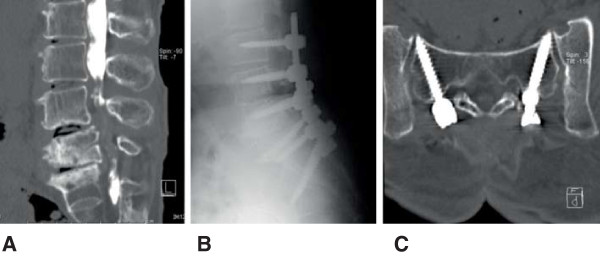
Radiographs of a 67-year-old female with degenerative kyphosis and spinal canal stenosis in the lumbar spine. A: Sagittal reconstruction image from the data of a computed tomography after the myelogram. B: Lateral view of the postoperative lumbar spine with instrumentation. C: Reconstructed image of the plane along the axes of intrasacral screws.
